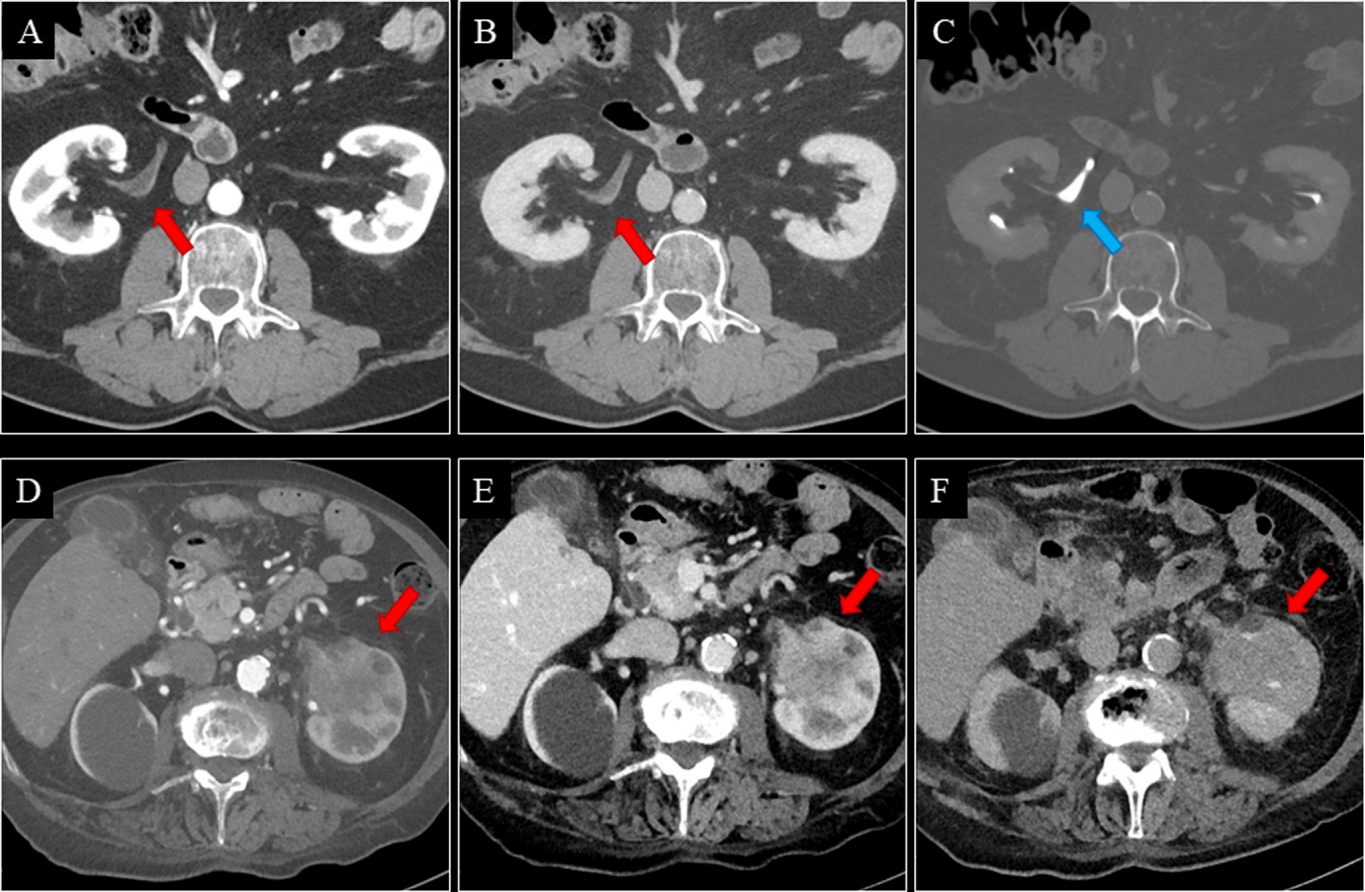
(A–C) A 68-yr-old male with a flat-growing pT1 tumour in the right renal pelvis. Both the control and the experimental CT were negative for renal pelvis UC in both reading teams. The tumour was detected at URS due to visible blood from the right ostium at cystoscopy. Retrospectively, a minimal contrast enhancement of the urothelium in the right renal pelvis (red arrow) was seen on axial CMP (A) and NP (B), but no filling defect was seen on axial EP (C; blue arrow). (D–F) An 87-yr-old male with a solid tumour in the left renal pelvis. Both the control and the experimental CT were positive for renal pelvis UC in both reading teams. The tumour (red arrow) was visible on all phases (axial CMP [D], NP [E], and EP [F]). The patient was unfit for URS, biopsy, and surgery, and received radiotherapy. CMP = corticomedullary phase; CT = computed tomography; EP = excretory phase; NP = nephrographic phase; pT = pathological tumour stage; UC = urothelial carcinoma; URS = ureterorenoscopy.
